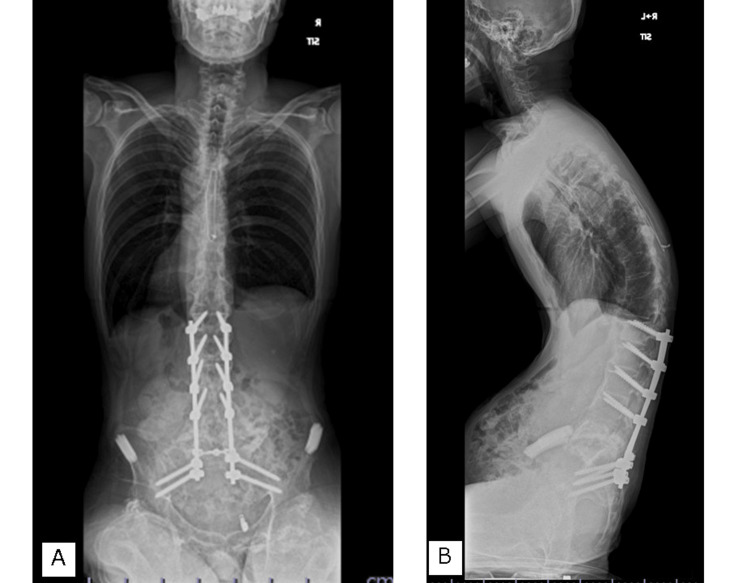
Final follow-up X-ray A. Anteroposterior X-ray with independent sitting; B. Lateral Xray with independent sitting
